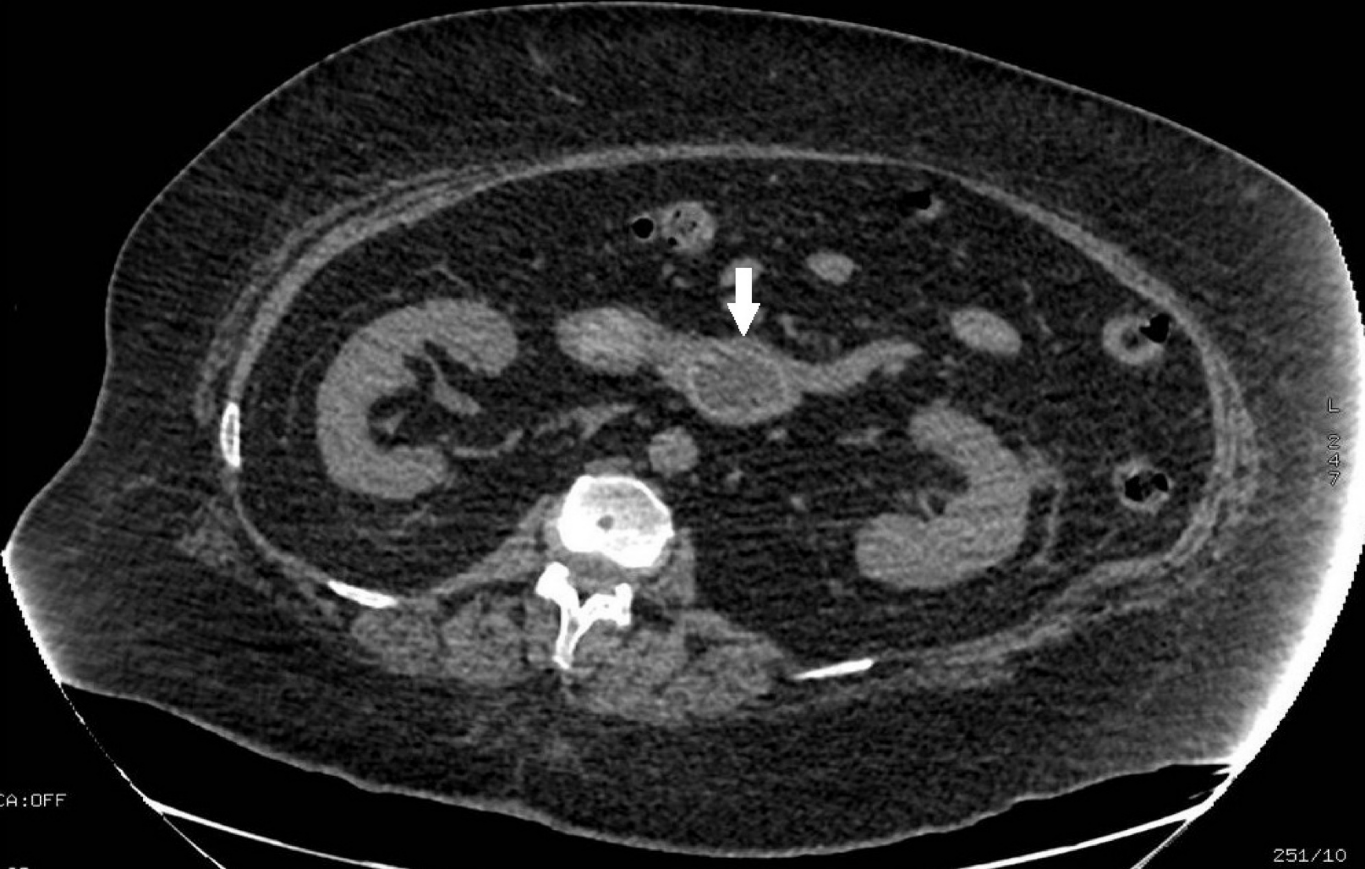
Abdominal CT scan axial cut showing the obstructing intraluminal stone in the duodenum (arrow) CT: computed tomography
